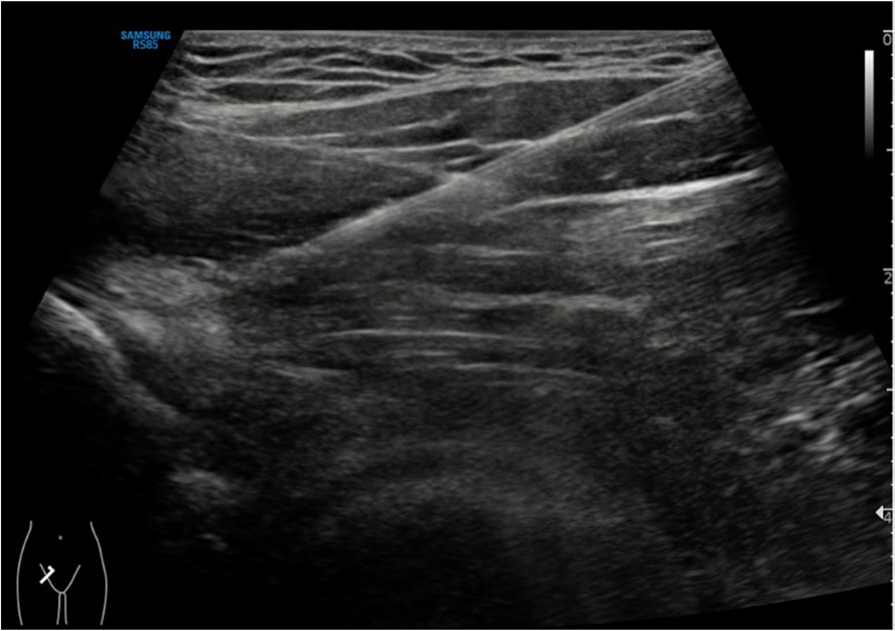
Imaging of Ultrasound Guided AIIS Injection
